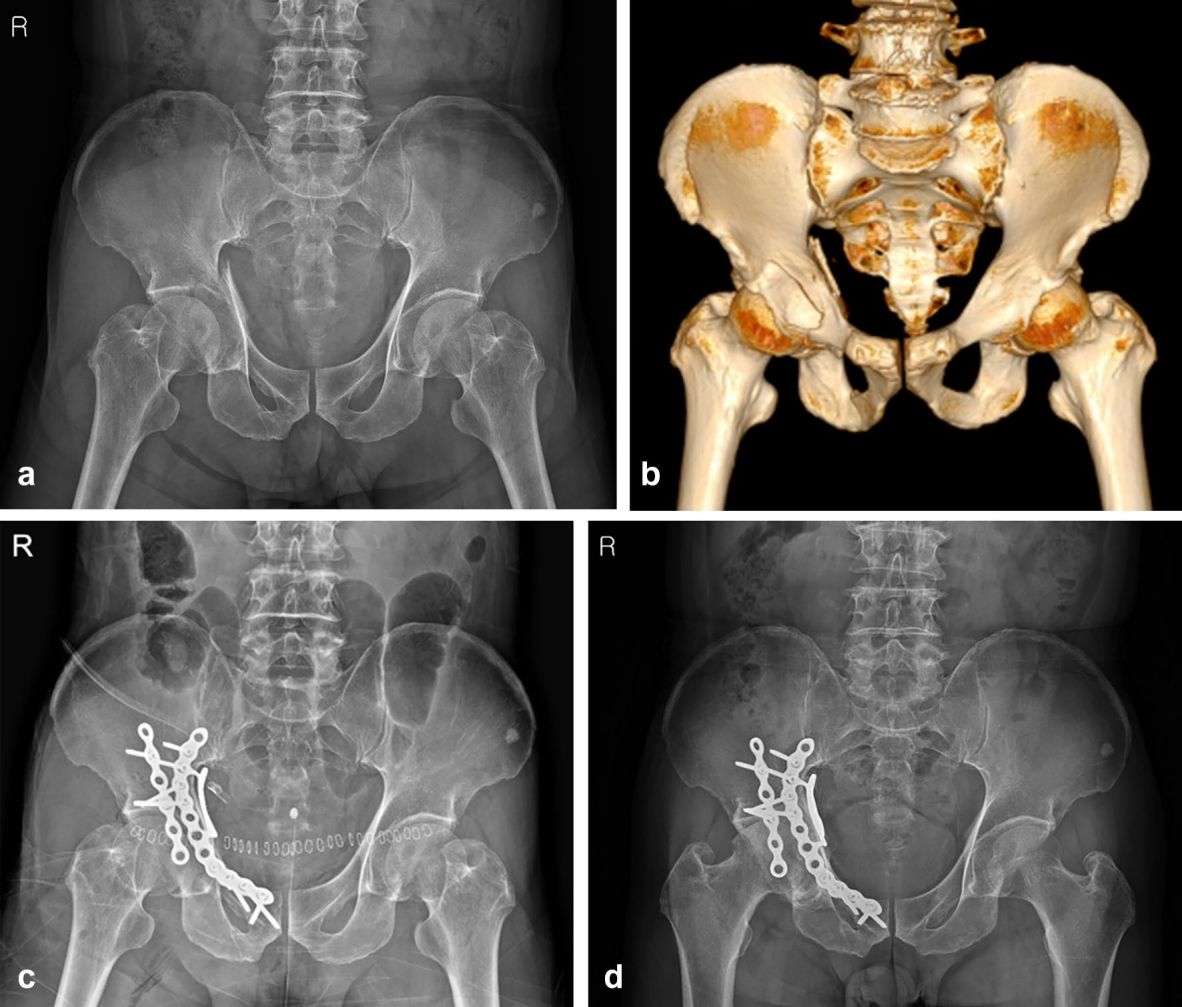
(a) pelvis anteroposterior radiograph and (b) 3D-CT of a 67-year-old male, superomedially displaced acetabular fracture with anterior wall fragment. (c) Immediate postoperative and (d) 18 months after surgery radiograph, after fixed with quadrilateral surface plate and additional buttress plate.
